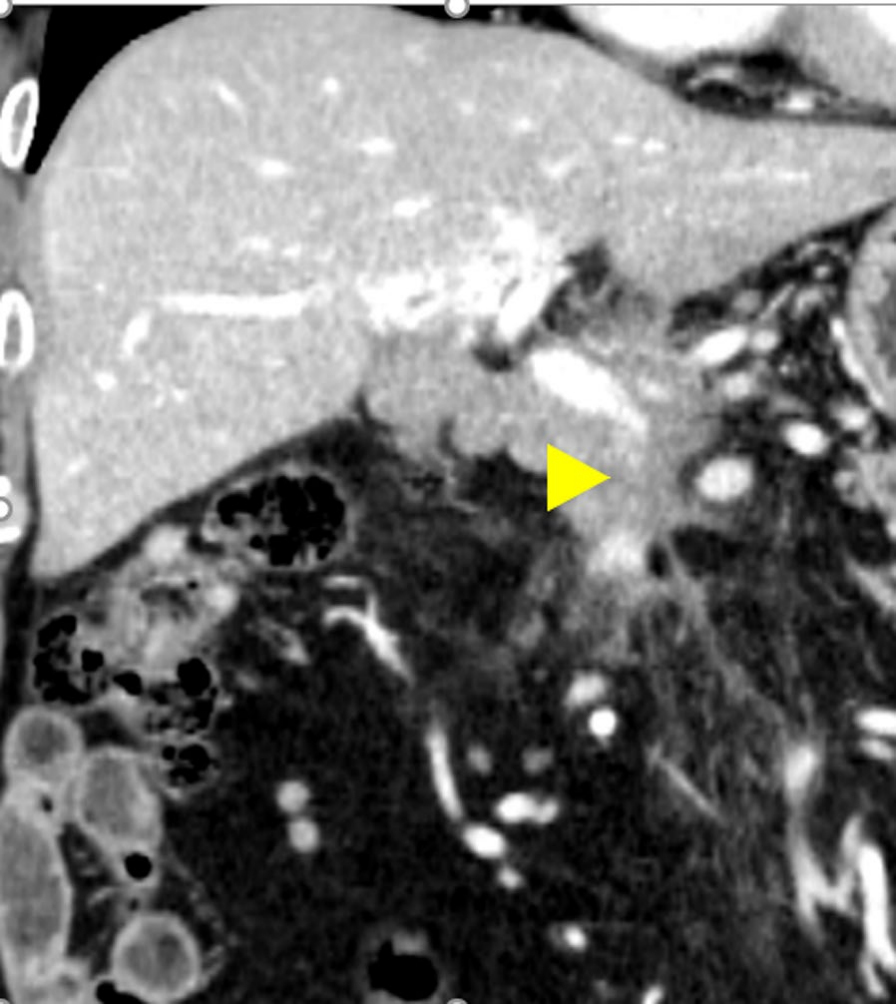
Follow-up computed tomography a year and 6 months after pancreaticoduodenectomy (coronal image). The superior mesenteric vein was obstructed near the splenic vein confluence (arrowhead)
